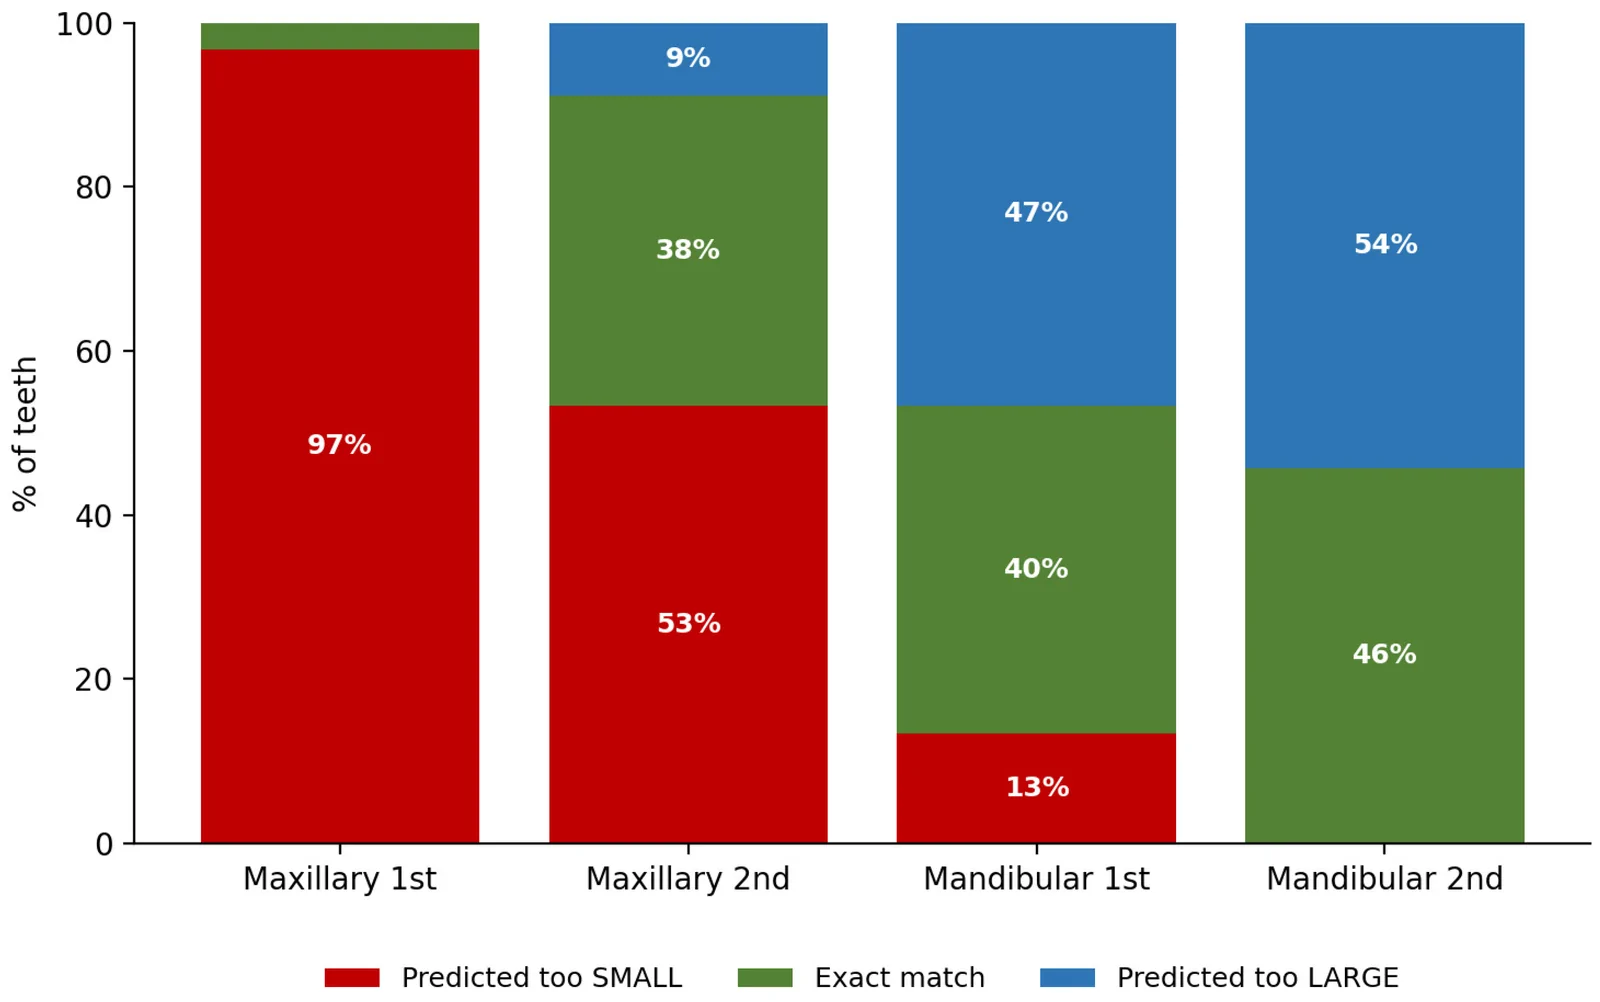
Direction of radiographic prediction error by tooth type.
